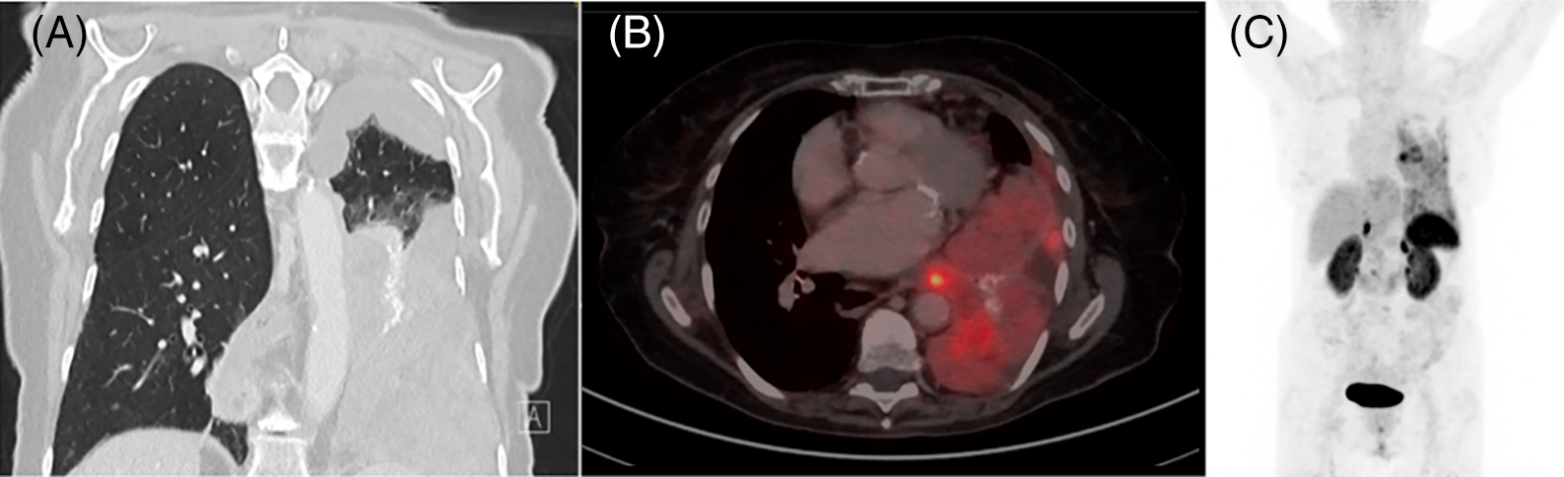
Imaging of the intrathoracic SFT before redebulking surgery. (A) Coronal chest‐CT showing a large heterogeneous tumor mass infiltrating the mediastinum, pleura, pericardium, and diaphragm. In addition, the expansion of the left lung is significantly reduced. (B, C) Transversal fusion (B) and maximum intensity projection (C) of [^68^Ga]DOTATATE/PET‐CT showing the SFT and further lesions in the pericardial fat tissue with variable and focally increased SSTR‐expression.
